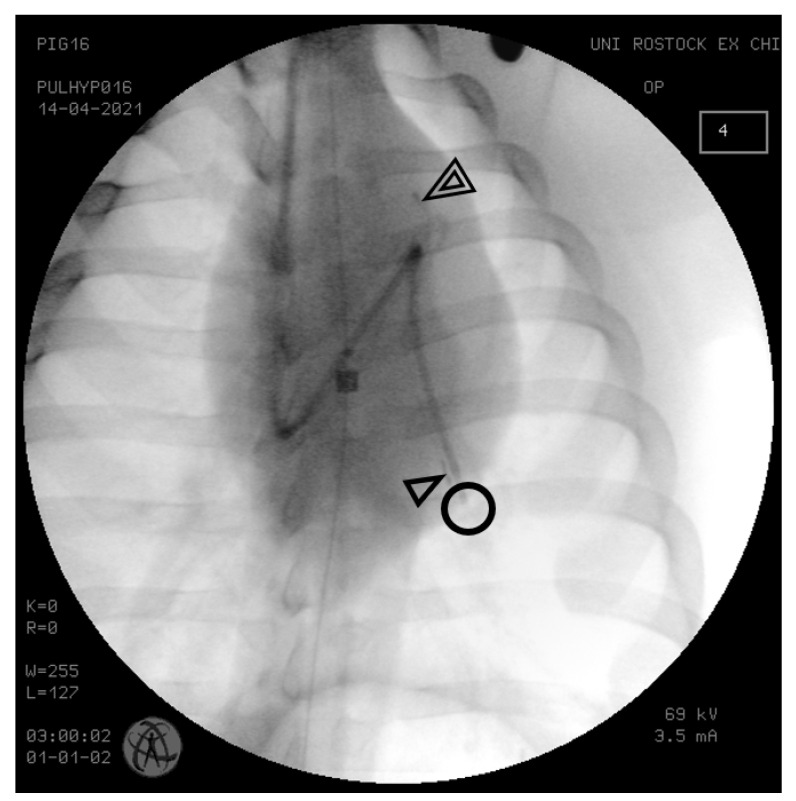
Thoracic X-ray to confirm correct positions of pulmonary artery catheters. A conventional pulmonary artery (Swan-Ganz) catheter was placed in the pulmonary artery for gold standard measurement of pulmonary artery pressure (circle). The tips of two Millar Mikro-Tip^TM^ pressure catheters were placed in a proximal (double open triangles) and in a distal branch of the pulmonary artery (open triangle).
